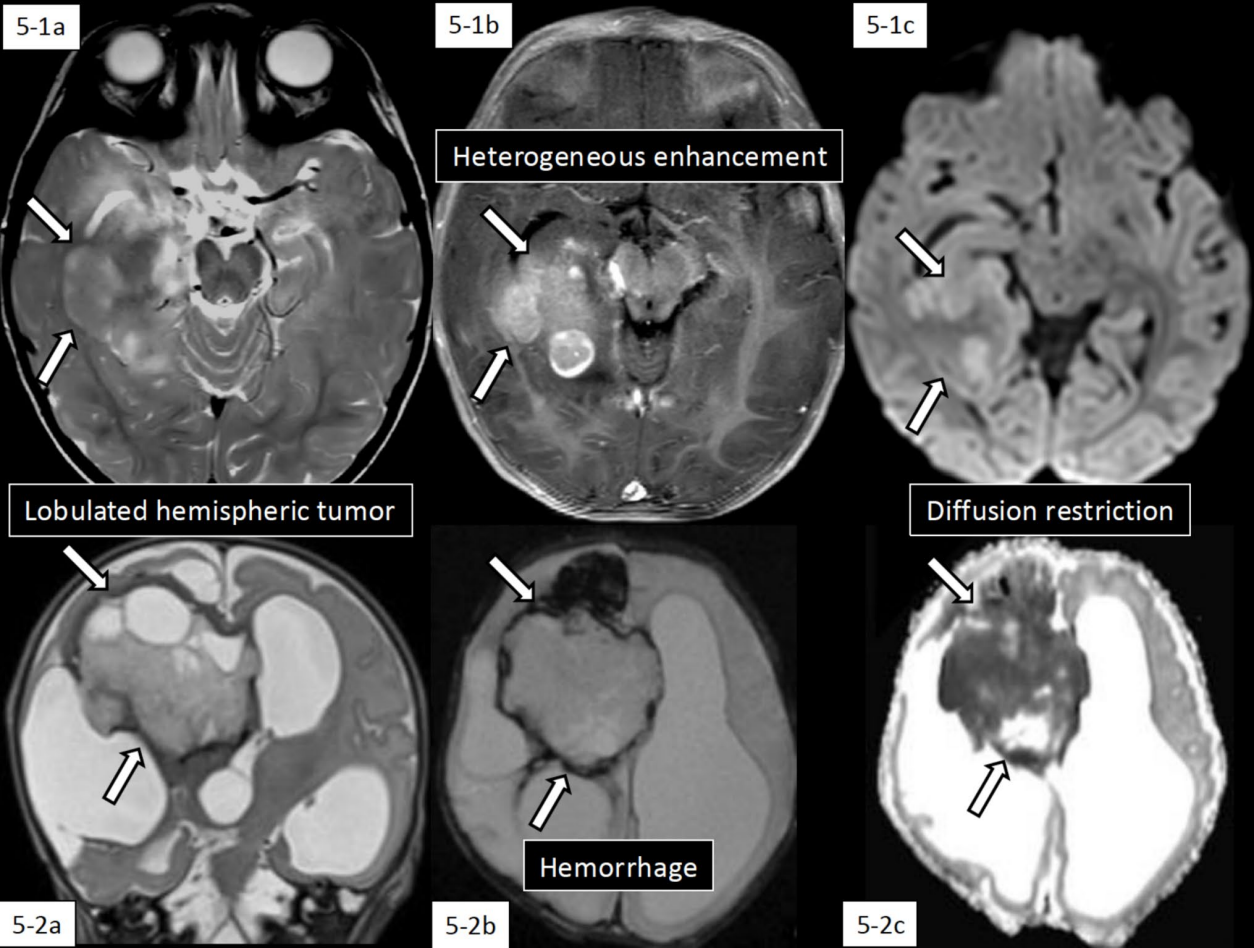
Imaging features of infant-type hemispheric glioma. 5-1 (10 months old, girl; STRN-NTRK2 fusion-positive): A lobulated tumor mass with heterogeneously hyperintense nodules on T2-weighted imaging (5-1a, arrows) and heterogeneous contrast enhancement on contrast-enhanced T1-weighted imaging (5-1b, arrows) with hyperintensity on diffusion-weighted imaging (5-1c, arrows) and low apparent diffusion coefficient (not shown). The same case with different images have been evaluated in our previous study [3]. 5-2 (a newborn; NTRK1 fusion-positive): A solid and cystic tumor mass obliterates the right foramen of Monro causing compression and contralateral displacement of the third ventricle and severe hydrocephalus (arrows). The tumor shows hyperintensity on T2-weighted coronal imaging (5-2a), hemorrhage on T2*-weighted imaging (5-2b), and low apparent diffusion coefficient indicating hypercellularity (5-2c). The images of this case was cited from Toniutti et al. [103] under a Creative Commons Attribution 4.0 International License
